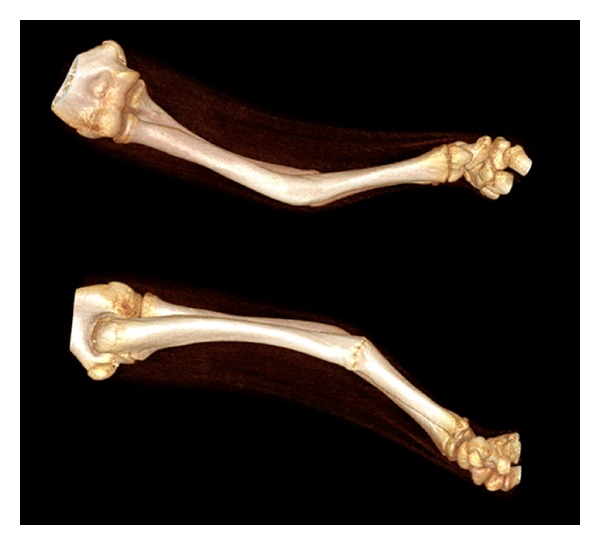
3D reconstructions of CT data showing the right forearm deformity.
